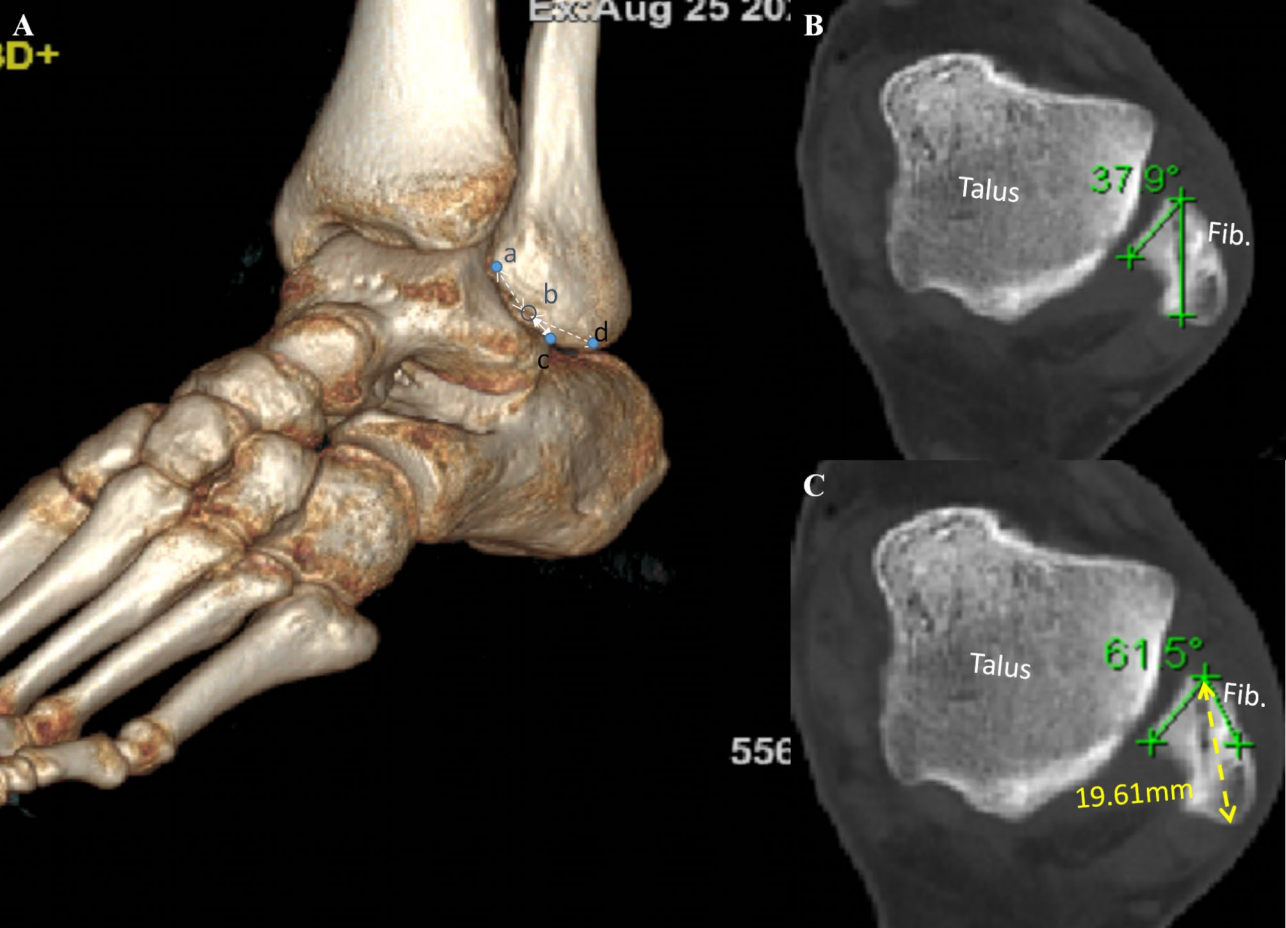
Postoperative 3d-CT scan. A: a: anteror fibular tubercle(AFT); b: bone tunnel; c: FOT; c: fibular tip; B,C: axial plain of CT scan, fib.: fibula
